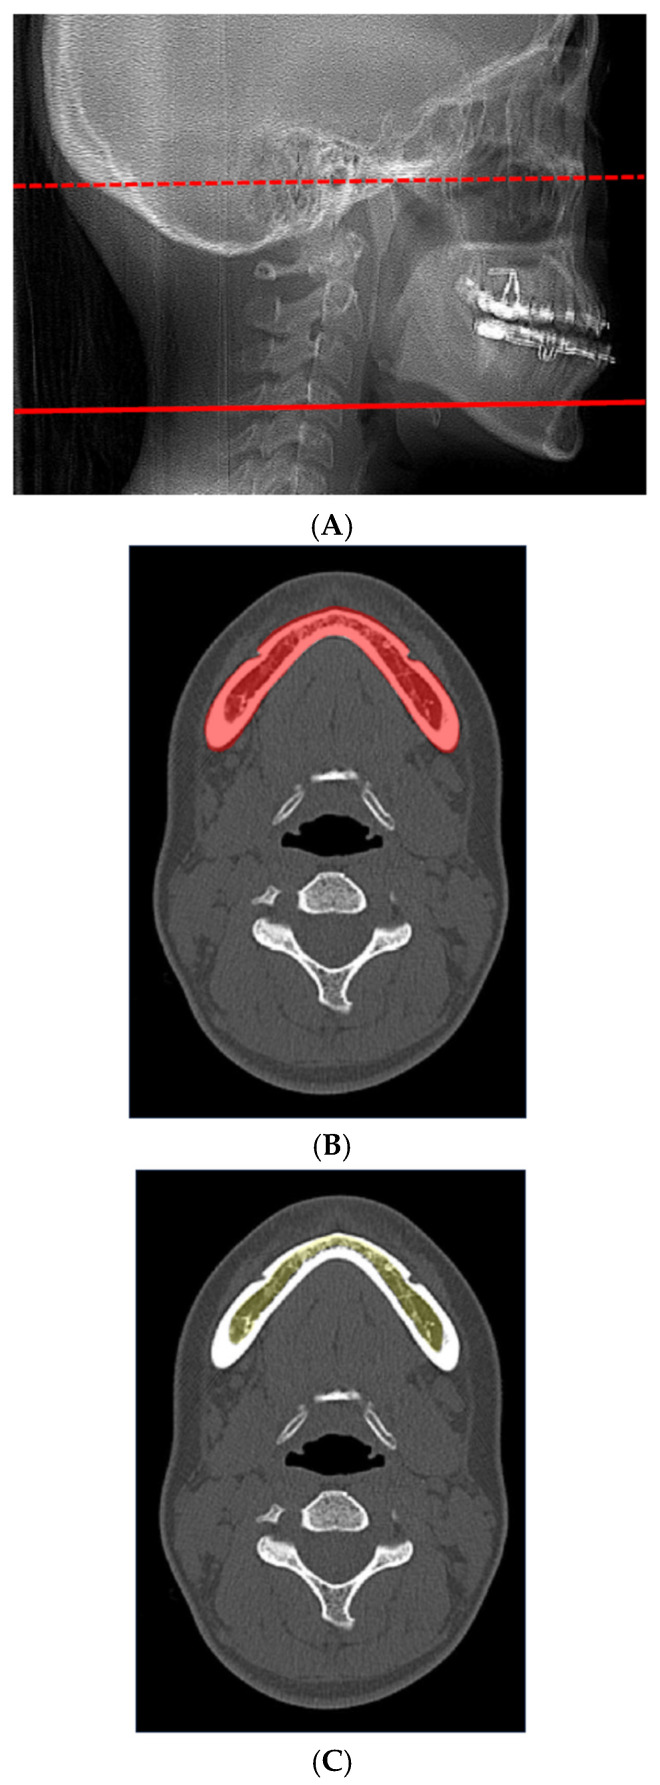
Mandibular body measurements. (A) Lateral CT for measurements of the mandibular body. FH plane, dotted line; measurement position, solid line. (B) Cortical and cancellous bone is extracted from axial-view CT of the mandibular body (red). (C) Cancellous bone is extracted from axial-view CT of the mandibular body (yellow). ROIs were placed in the mandibular body, including cortical (outer layer) and cancellous (trabecular) bone, avoiding soft tissues and teeth. A slice parallel to the FH plane, including the mental foramen, was used.
